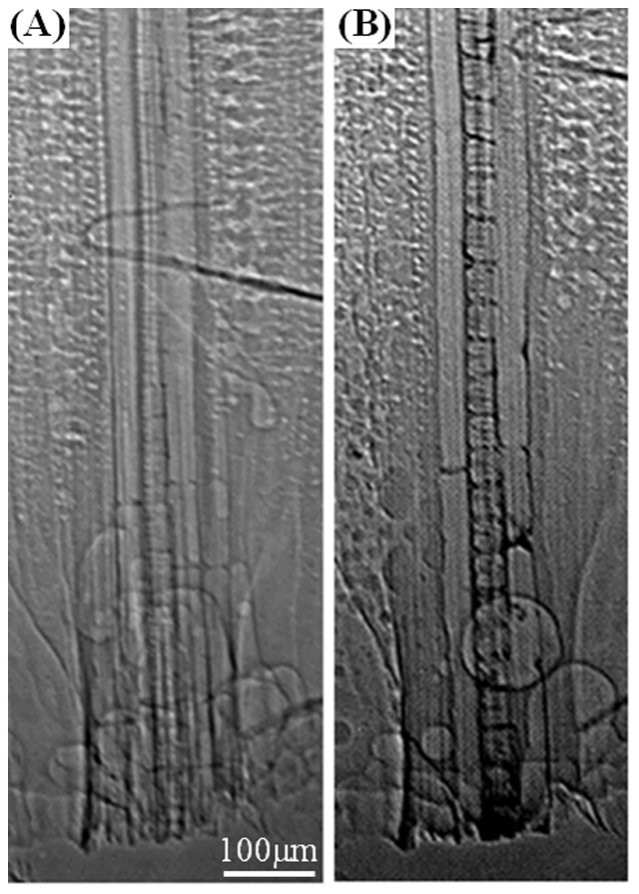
Typical X-ray images of xylem vessels in a maize leaf. Identical xylem bundle (A) before the first rehydration with AuNP solution and (B) after the first rehydration and dehydration cycle. Vessel wall is darkened by attached AuNPs.
